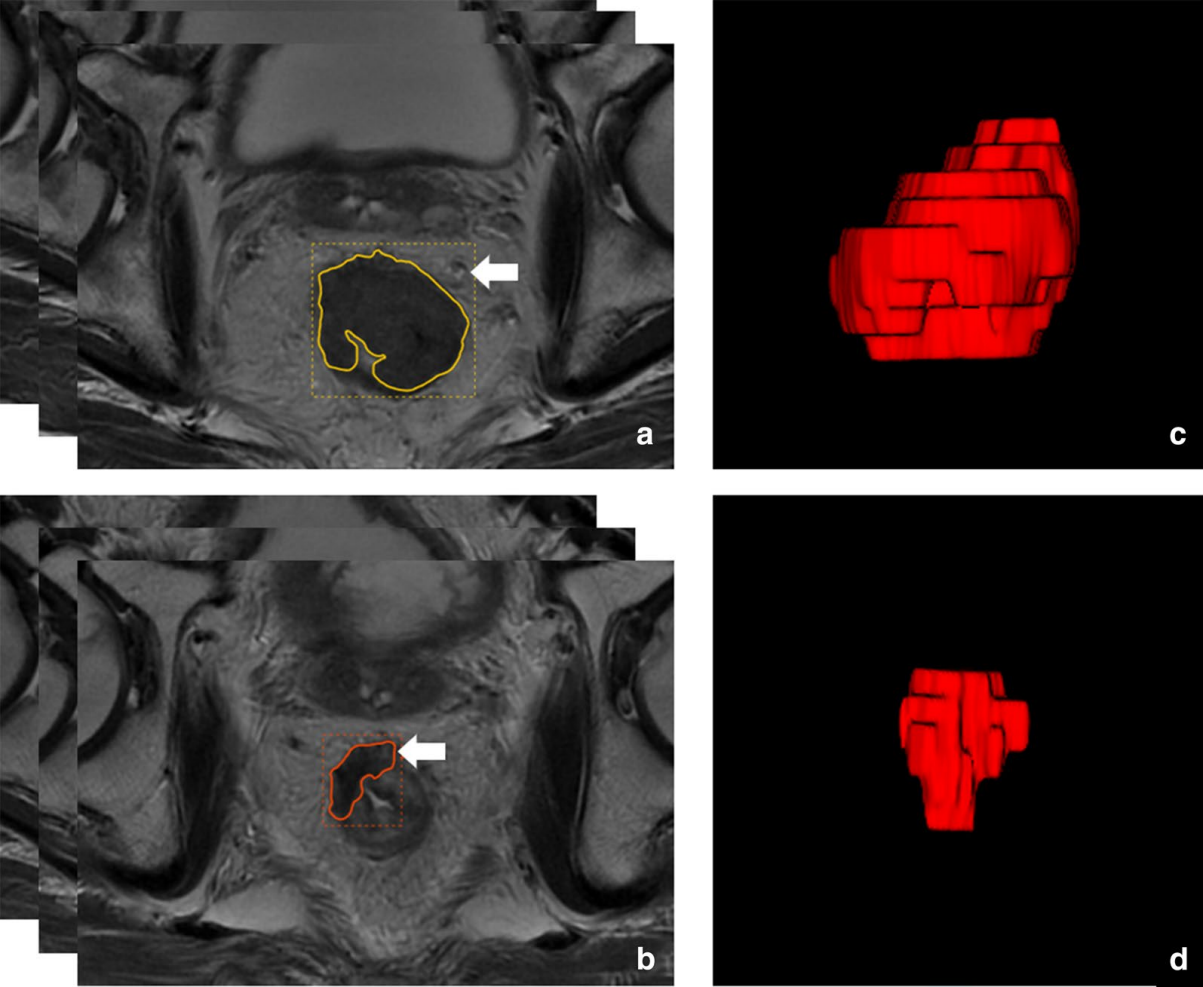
Representative images for lesion contouring. a–d. Images acquired in a 54-year-old man with LARC, staged as cT3N0. He underwent MRI scanning pre- and post- nCRT. a, b Delineation of ROIs on oblique-axial T2-weighted MR images pre- and post- nCRT (arrow). c, d Volume renderings of VOIs pre- and post- nCRT
